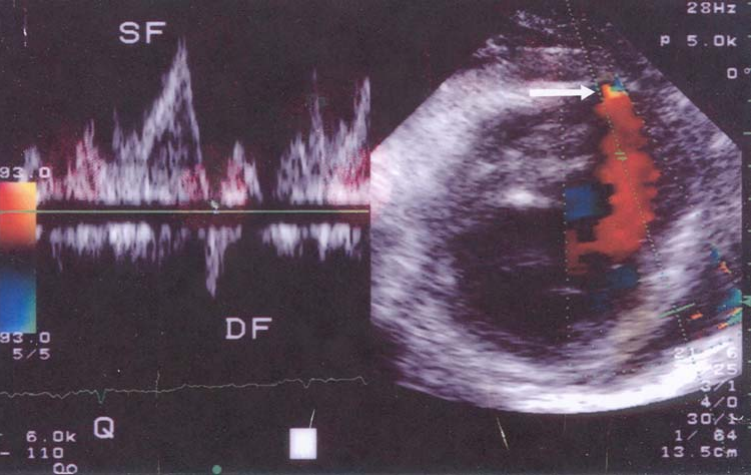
Pulsed wave Doppler showing a systolic flow (SF) from the LV cavity to the pseudoaneurysm and a diastolic regurgitant flow (DF) in the opposite direction. In the right part of the picture, colour Doppler depicts a flow between right and left ventricle (white arrow).
